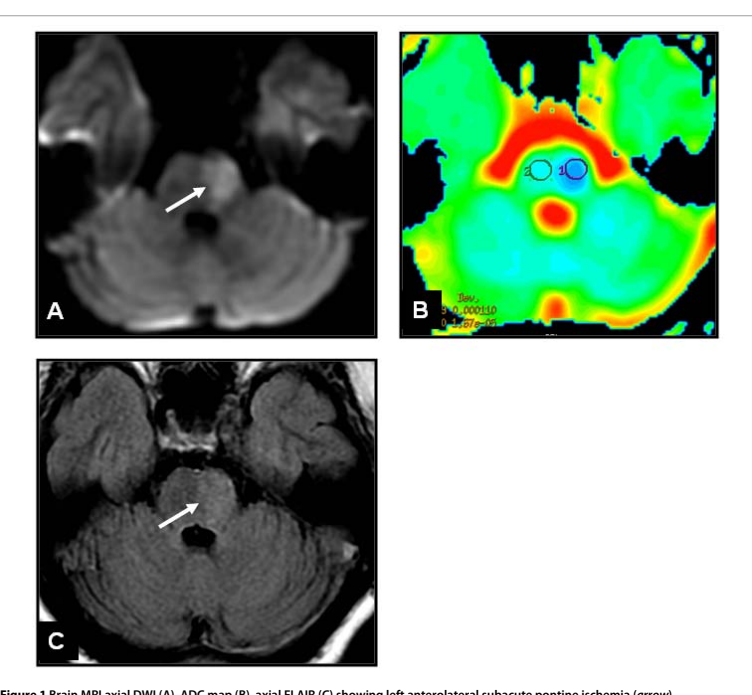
Brain MRI axial DWI (A), ADC map (B), axial FLAIR (C) showing left anterolateral subacute pontine ischemia (*arrow*).
